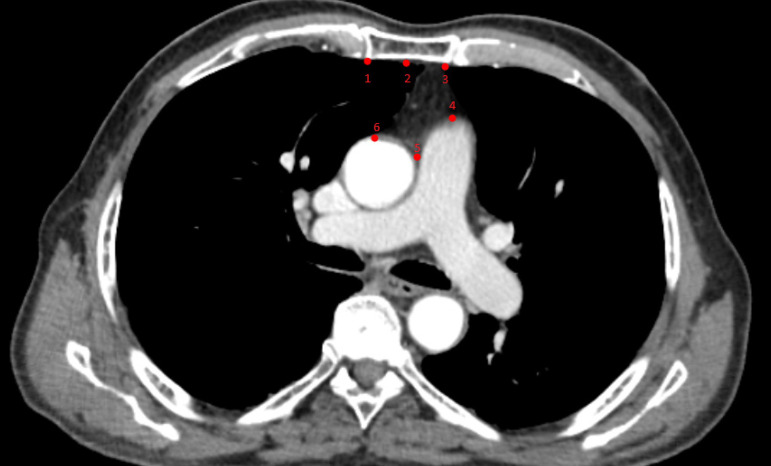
A computed tomography scan showing landmarks markings on retrosternal space at the main pulmonary artery branching level.
